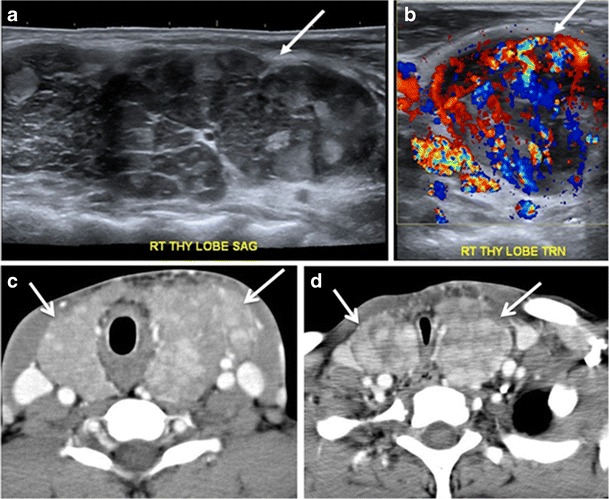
A 19-year-old male patient known to have multi-nodular goiter and FNA, showing underlying Hashimoto’s thyroiditis. a, b Sagittal and transverse greyscale and colour Doppler ultrasound of the neck demonstrate a hypoechoic enlarged right thyroid lobe with small hyperechoic regenerative nodules and marked hypervascularity (*white arrows*). c, d Enhanced axial CT scan images of the neck demonstrate a heterogeneously enhancing and enlarged thyroid gland, left more than right lobe, and the trachea is markedly narrowed
